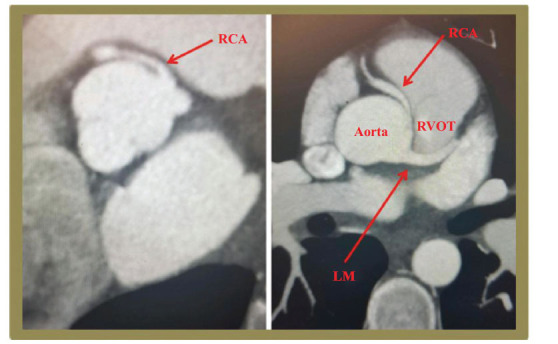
CT Angiogram showing the right coronary artery (RCA) originating from the left coronary cusp adjacent to the left main coronary artery (LMCA). Reproduced with kind permission from Prof Bassam Omar: Kolakalapudi P, Sachdev S, Omar B. Anomalous Coronary! Is It Time To Panic! Cardiofel Newslet 2019; 2: 13-14.
